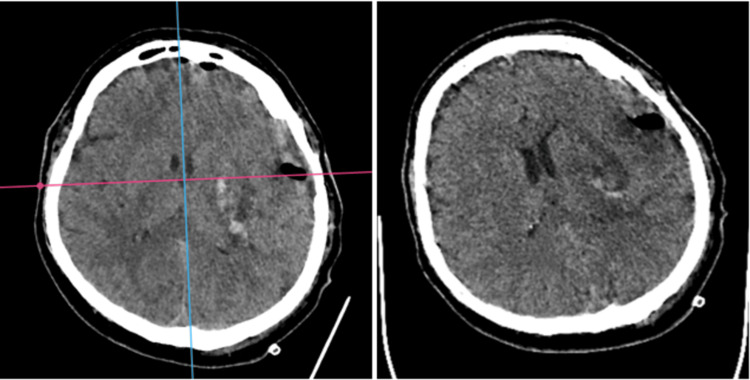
Postoperative cranio-cerebral CT obtained during follow-up evaluations A 24-hour postoperative CT scan (axial view) shows minimal residual hematoma with no recurrence of hemorrhage; no midline shift or mass effect is noted.
